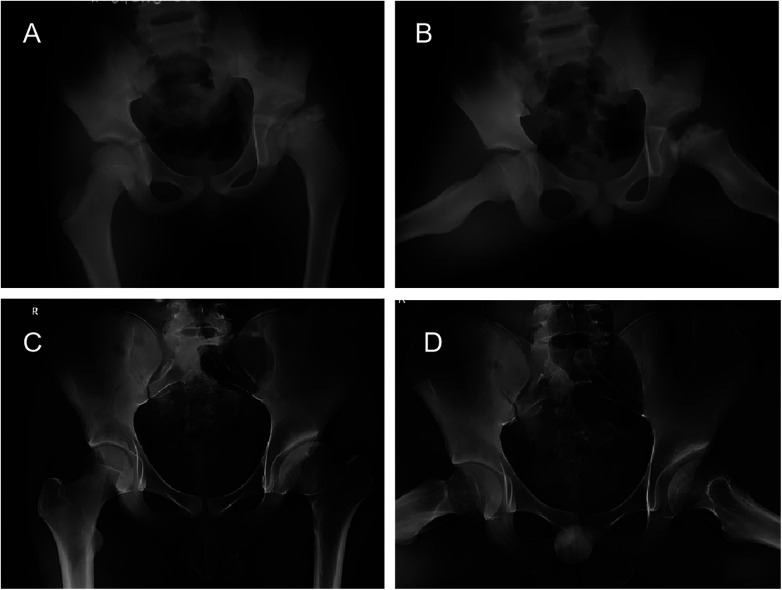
Serial radiographs of a 7-year-4-month-old boy with left femoral head necrosis in the early reossification stage who received conservative treatment. (A) Anteroposterior pelvic radiograph at initial presentation; the affected hip was the left hip. (B) Frog-leg lateral radiograph at initial presentation of the left hip. (C) Later follow-up radiograph showing persistent deformity of the left femoral head and acetabular changes. (D) Radiograph at the final follow-up of 8 years, showing that the acetabular inclination angle on the affected (left) side was markedly greater than that on the contralateral side; the outcome was classified as Stulberg grade III (class II).
